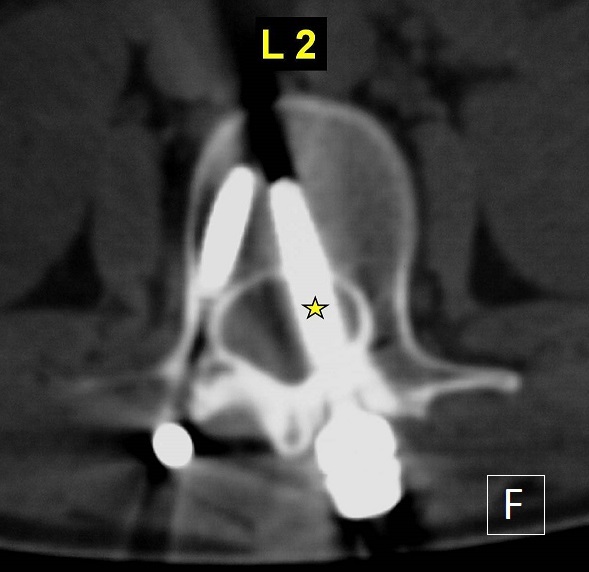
Axial CT-scan (bony window) through the second lumbar vertebrae showing the malpositioned left pedicle screw into the spinal canal (star)
